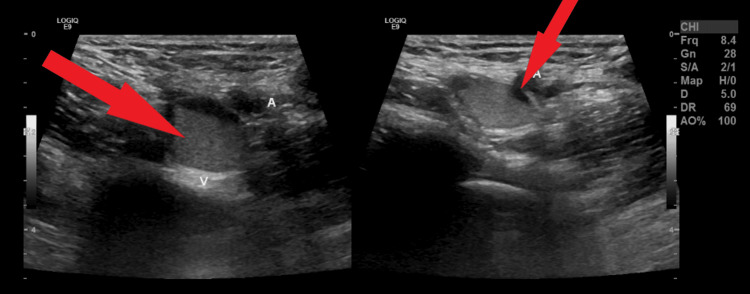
Ultrasound of the left lower extremity showing deep vein thrombosis (DVT), indicated by the red arrows
